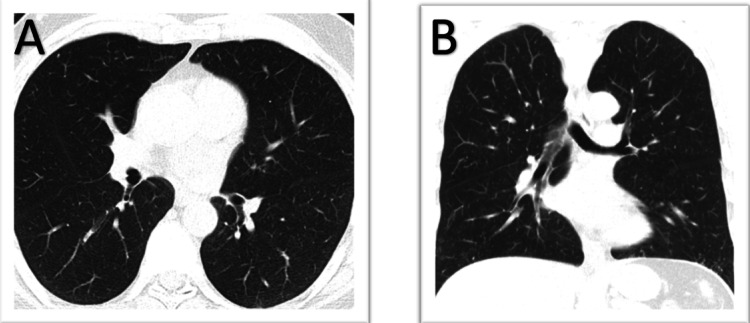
Baseline chest CT (A) axial and (B) coronal views showing subcentimeter pulmonary nodules in the anterior right and left upper lobes with reticulonodular changes in bilateral lower lobes
